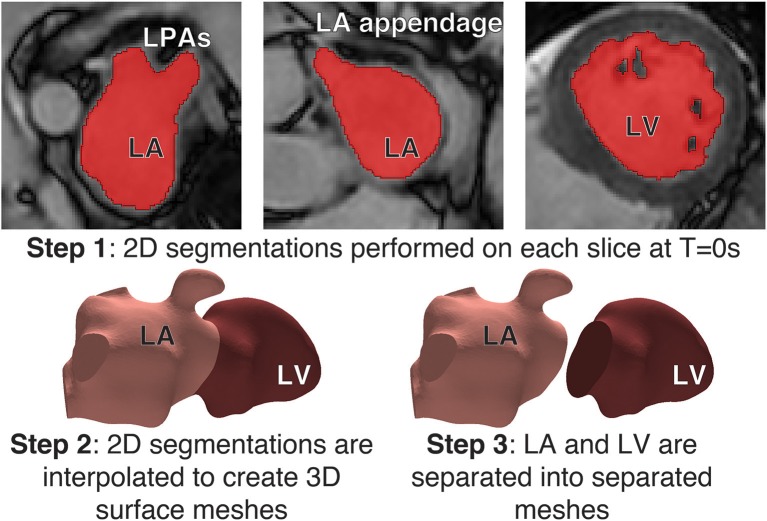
Segmentation process: Step 1: (Top) Segmentations of the LA and LV were performed with the image processing package MITK on each Cine MRI dataset at Time = 0s to create a patient-specific 3D atrial and ventricle mesh. Segmentations were performed on each slice in the axial plane. Step 2 (Bottom-left) A 3D interpolation is applied to the segmentation to create a smooth surface mesh. Step 3: (Bottom-right) the mesh is separated into the LA and LV at the mitral valve.
